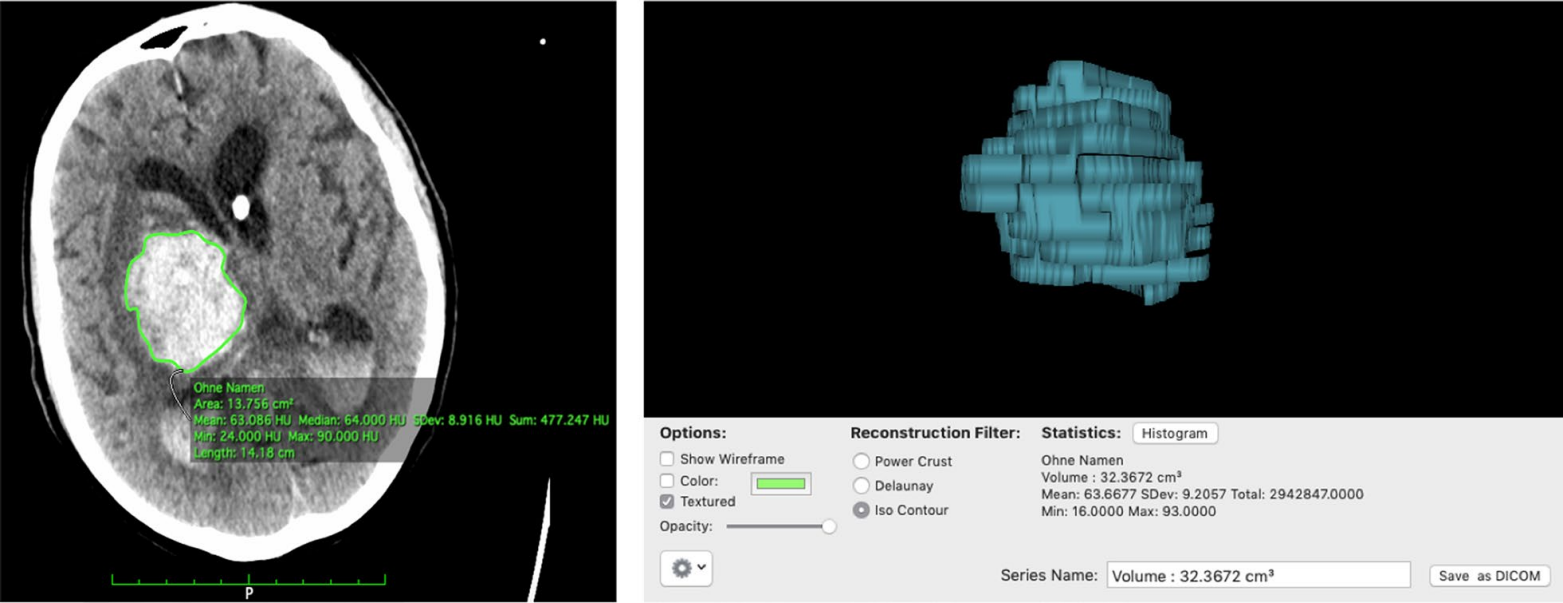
Example for the region of interest (ROI) volumetry using OsirixLite (Pixmeo SARL, Switzerland). Axial CT scans were checked for slides showing hyperdensity relating to ICH, and hematoma outlines were marked on corresponding slides (left). Hematoma volume was then calculated automatically using ROI volumetry function of the software (right)
